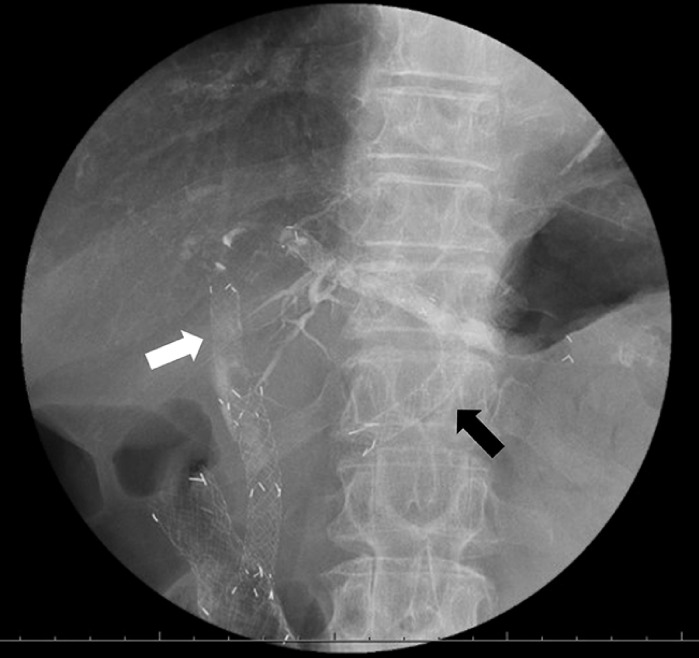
Fluoroscopic image showing endoscopic reintervention for recurrent biliary obstruction. Because the partially covered self-expandable metal stent (PCSEMS) inserted for endoscopic ultrasound-guided hepatogastrostomy (EUS-HGS) was not removed, an additional SEMS (black arrow) was deployed through the stent mesh of the EUS-HGS PCSEMS, with an additional antegrade stent placed across the malignant biliary obstruction (white arrow).
